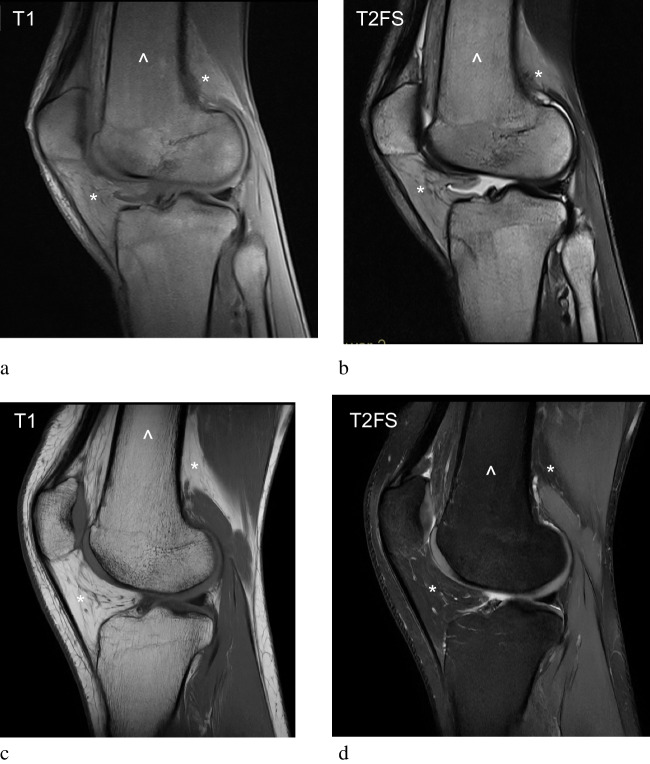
SABM in a 53-year-old female with a body mass index (BMI) of 16 kg/m^2^ due to excessive exercise. Sagittal T1 weighted (a) and T2 fat-saturated (T2 FS) (b) sequences of the knee demonstrate diffuse hypointense marrow signal on T1 and mild hyperintensity on T2 FS (arrowheads). Characteristic signal abnormalities are also demonstrated in the adjacent subcutaneous tissues (asterisks), with hypointense signal on T1-weighted images and hyperintense signal on fluid-sensitive sequences, giving rise to the inverted signal characteristics of SABM. Sagittal T1 weighted (c) and T2 FS (d) sequences of a normal knee for comparison, demonstrating normal marrow signal (arrowheads) and subcutaneous fat (asterisks)
